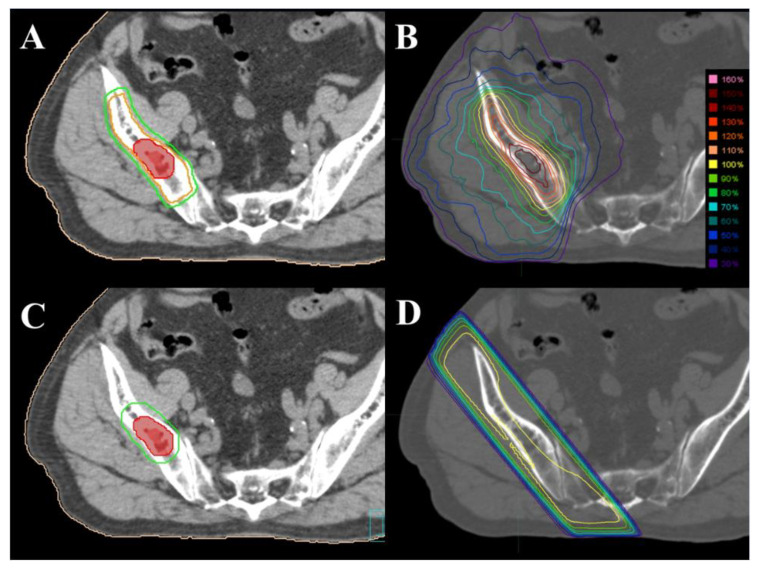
Axial computed tomography images with contours (red = gross tumor volume, orange = clinical target volume, and green = planning target volume) and dose distribution of stereotactic body radiotherapy (A,B) and conventional external beam radiotherapy (C,D) for coxal bone metastases.
